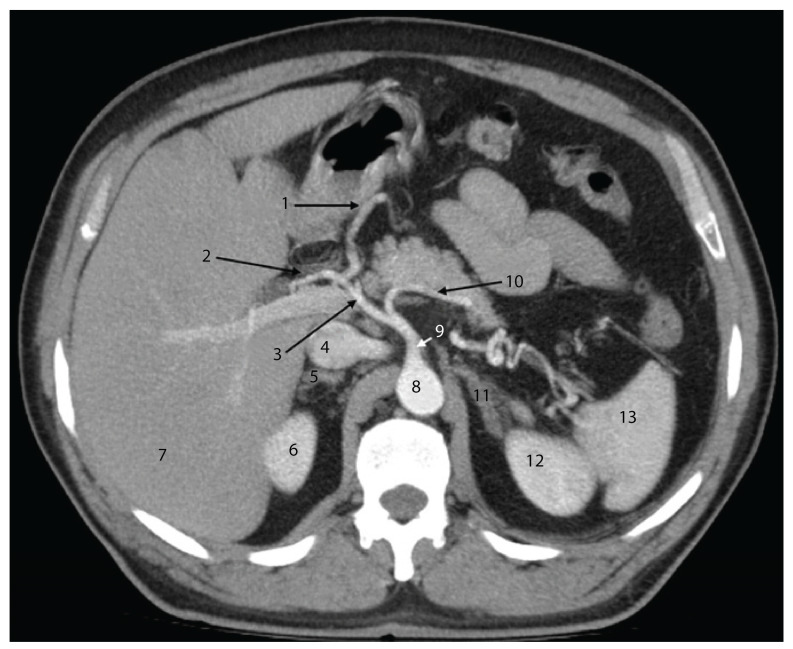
Visualization of the celiac trunk in an axial CT section during the arterial phase (MIP reconstruction with 4 mm slice thickness). 1—Right gastric artery; 2—proper hepatic artery; 3—common hepatic artery; 4—inferior vena cava; 5—right adrenal gland; 6—superior pole of the right kidney; 7—right hepatic lobe; 8—abdominal aorta; 9—celiac trunk; 10—splenic artery; 11—left adrenal gland; 12—superior pole of the left kidney; 13—spleen.
